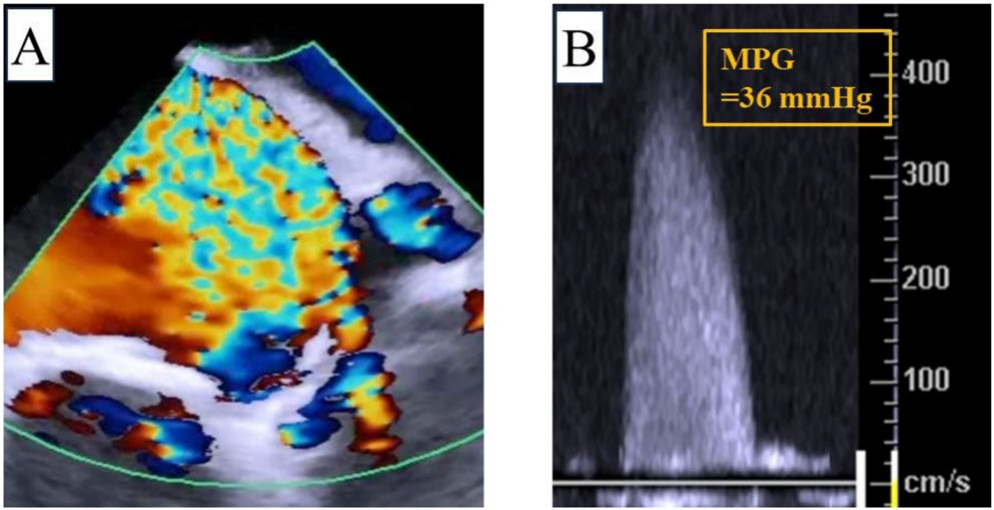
Preoperative assessment of aortic valve. (A) Color Doppler flow in long‐axis aortic valve view. (B) Continuous‐wave Doppler across the aortic valve. The aortic valve mean pressure gradient was 36 mmHg. MPG, mean pressure gradient.
